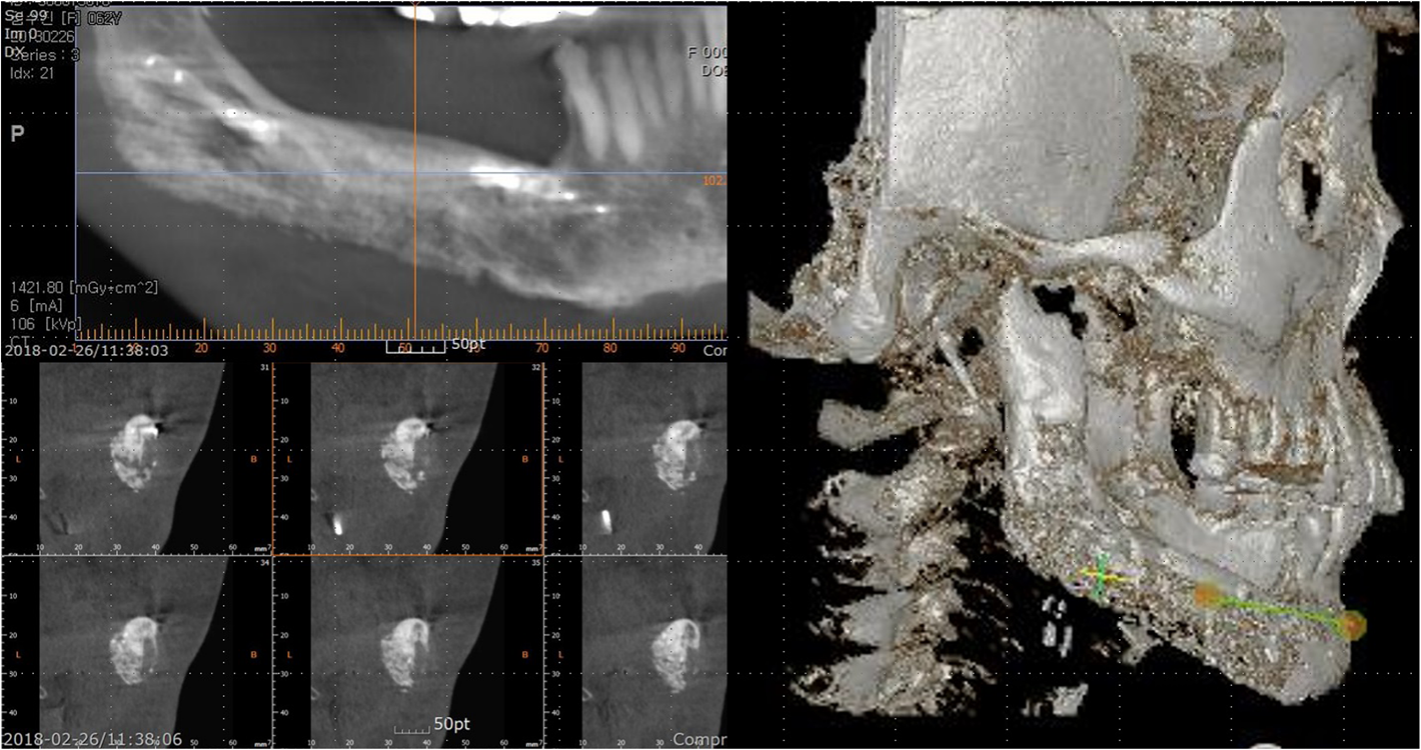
Neomandible body on CT view
